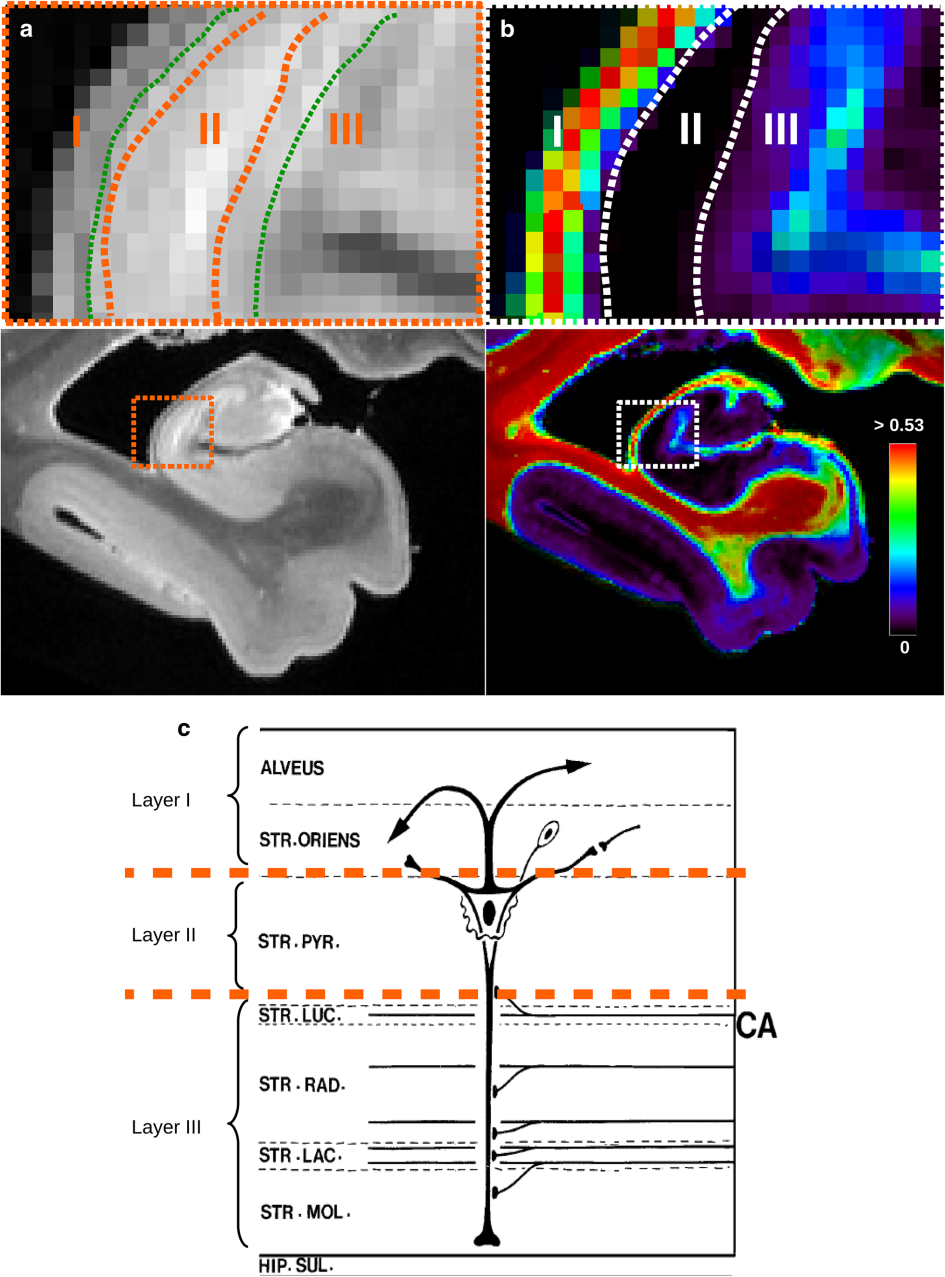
Comparison of the contrast between the three hippocampal layers using a a standard anatomical $$T_{2}$$-weighted spin-echo sequence and b the intra-cellular volume fraction map obtained with NODDI model. The heat scale represents the intra-cellular volume fraction. The theoretical layers in cornu Ammonis are drawn in c, adapted from Duvernoy (2005). Their boundaries have been recognized, based on the T2-weighted image, with the green dotted lines in a and also with the help of the intra-cellular volume fraction map as shown with the orange dotted lines in a and the white dotted lines in b
